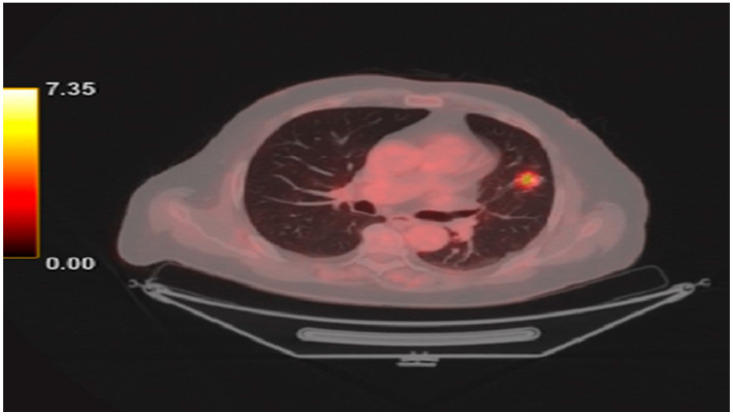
PET/CT demonstrating a hypermetabolic lesion in the glottic region, corresponding to the primary site of disease.
